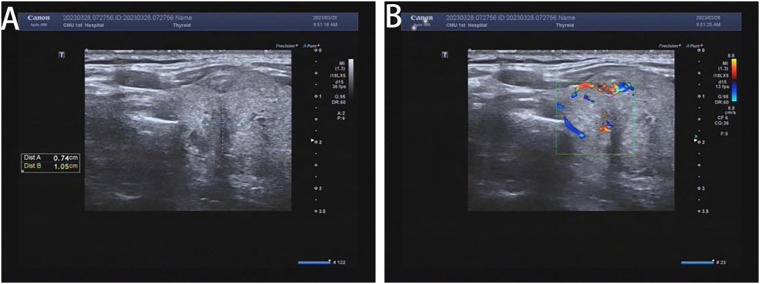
Thyroid ultrasound: (A) shows unclear boundaries and irregular shape in the right lobe of the thyroid gland with low echogenicity mass. (B) Shows punctate blood flow within the thyroid gland mass.
